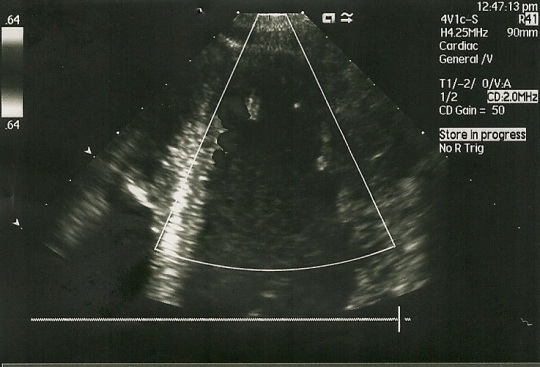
Echocardiography: LV magnified view of trabeculae
